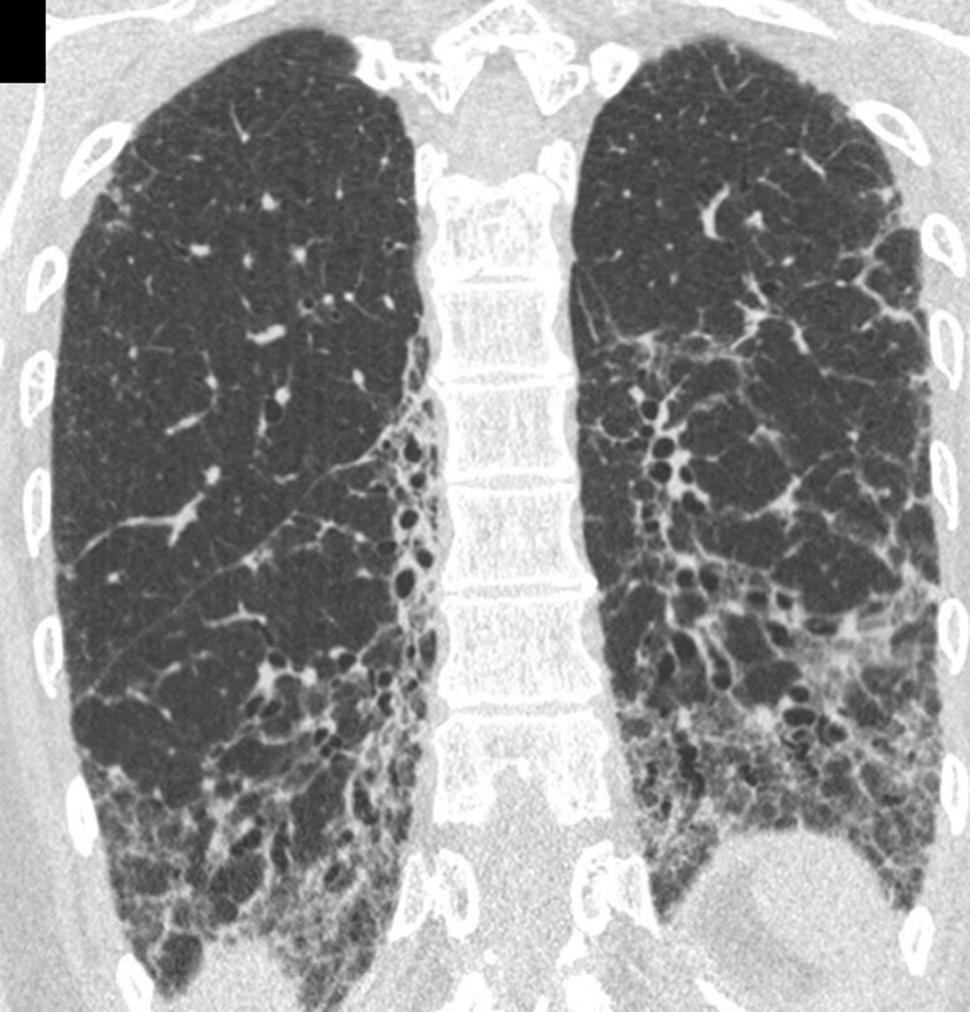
Fibrotic NSIP pattern. The multiplanar coronal reconstruction of this CT scan demonstrates extensive bilateral ground glass opacities with traction bronchiectasis with a peripheral and basal distribution
